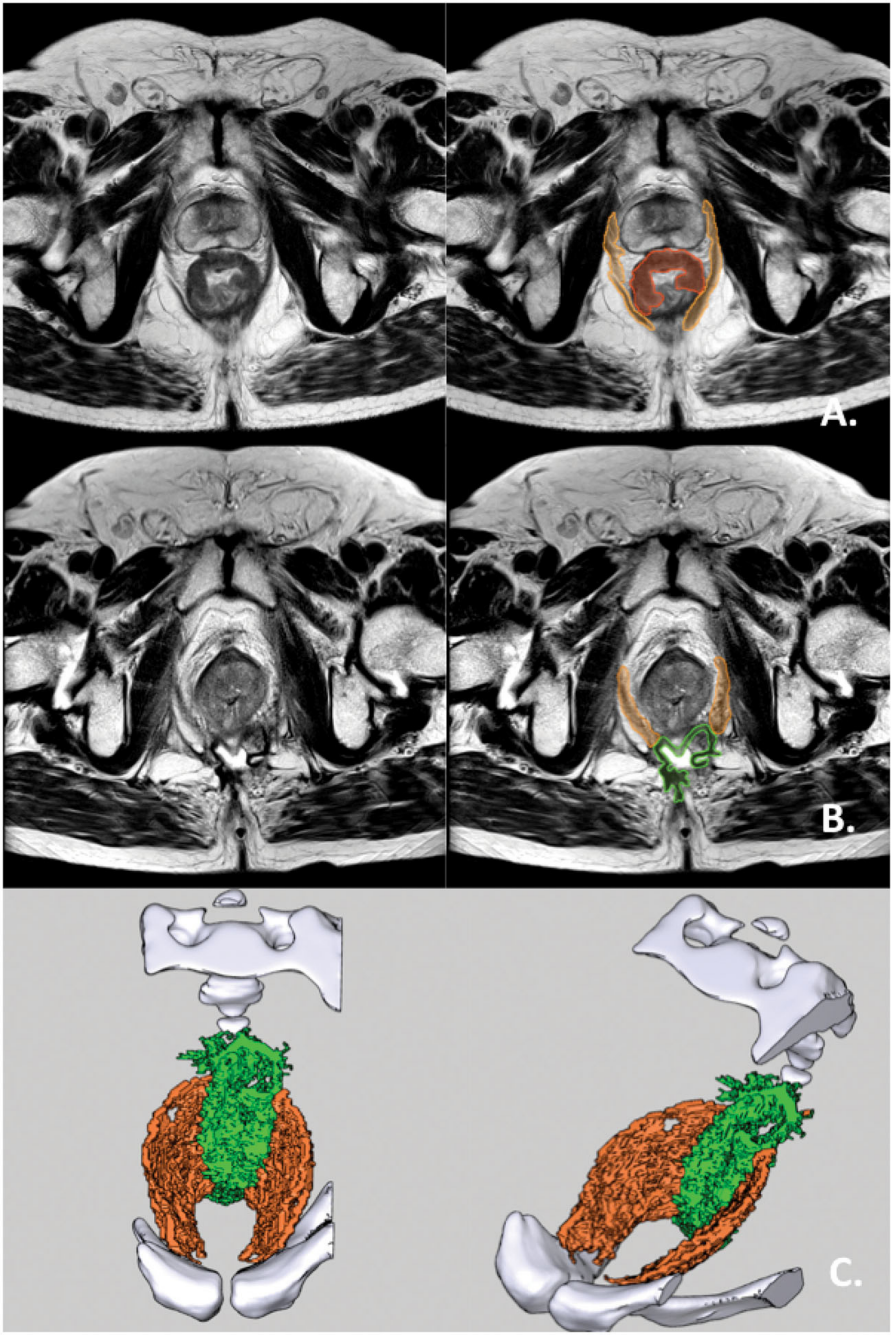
ELAPE with RPD in both posterior quadrants. (A) Preoperative axial T2-weighted MRI of a rectal cancer patient with an mrT3 tumor with invasive growth from 9 o'clock to 2 o'clock. The pelvic diaphragm is hatched in orange. The tumor is hatched in red. (B) Postoperative axial T2-weighted MRI of the same patient. RPD hatched in orange while supporting mesh hatched in green. (C) The pelvic diaphragm of the same patient, 3D-rendered and shown in superior and antero-lateral superior views. The rendering is made from the above postoperative MRI. The bony pelvis is rendered in white. RPD is rendered in orange. Supporting mesh is rendered in bright green.
